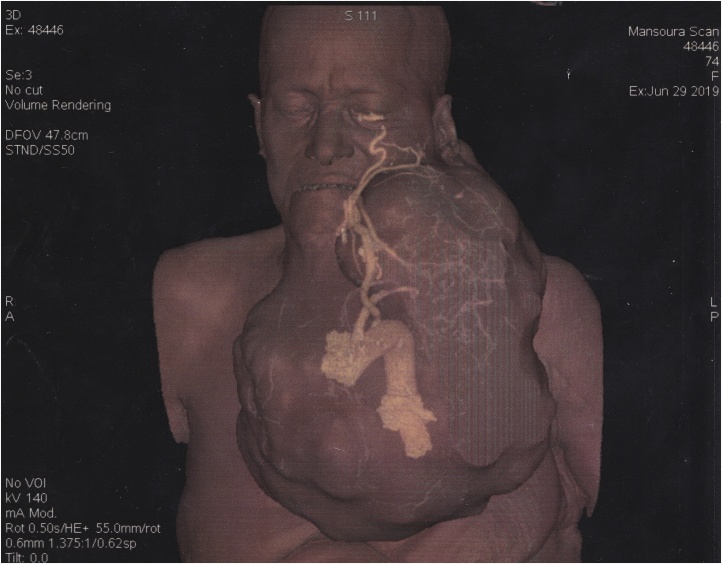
CT angiography (antero-posterior view).
